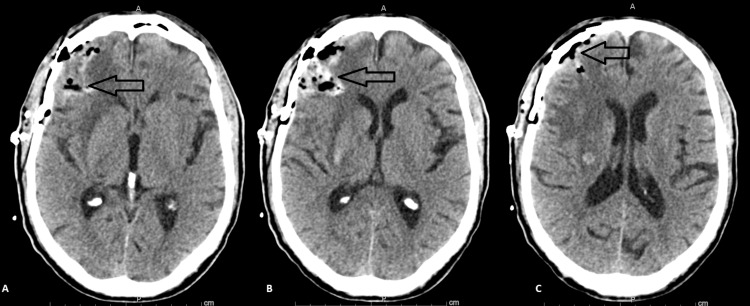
Post-Craniotomy CT Head Post-craniotomy CT head with a residual defect after resection (arrows in A, B, and C)
